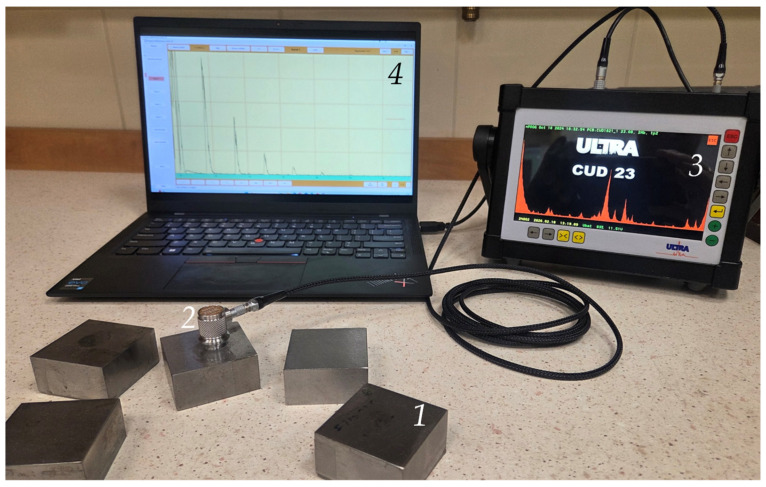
Test setup: 1—coated sample, 2—ultrasonic probe, 3—ultrasonic flaw detector, 4—computer (signal analysis).
